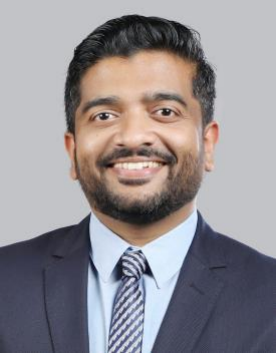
Dr Salman Salahuddin works as an interventional cardiologist at Aster MIMS Hospital, Calicut, India. He leads the Department of Adult Interventional Cardiology. An interventional cardiologist with expertise in complex coronary interventions, structural heart disease, and advanced echocardiography, he is actively involved in teaching, research, and improving patient outcomes.
